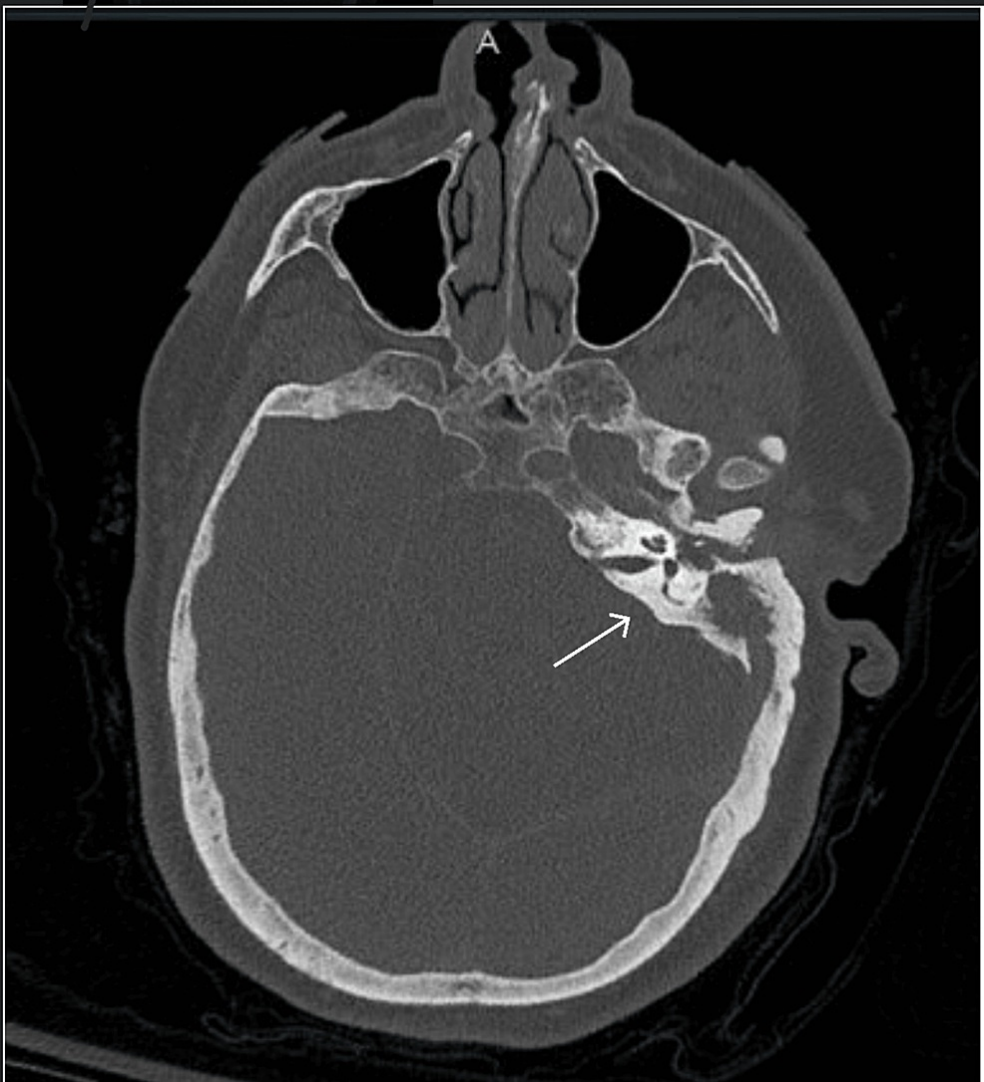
CT head revealing acute left-sided mastoiditis CT: Computed tomography
